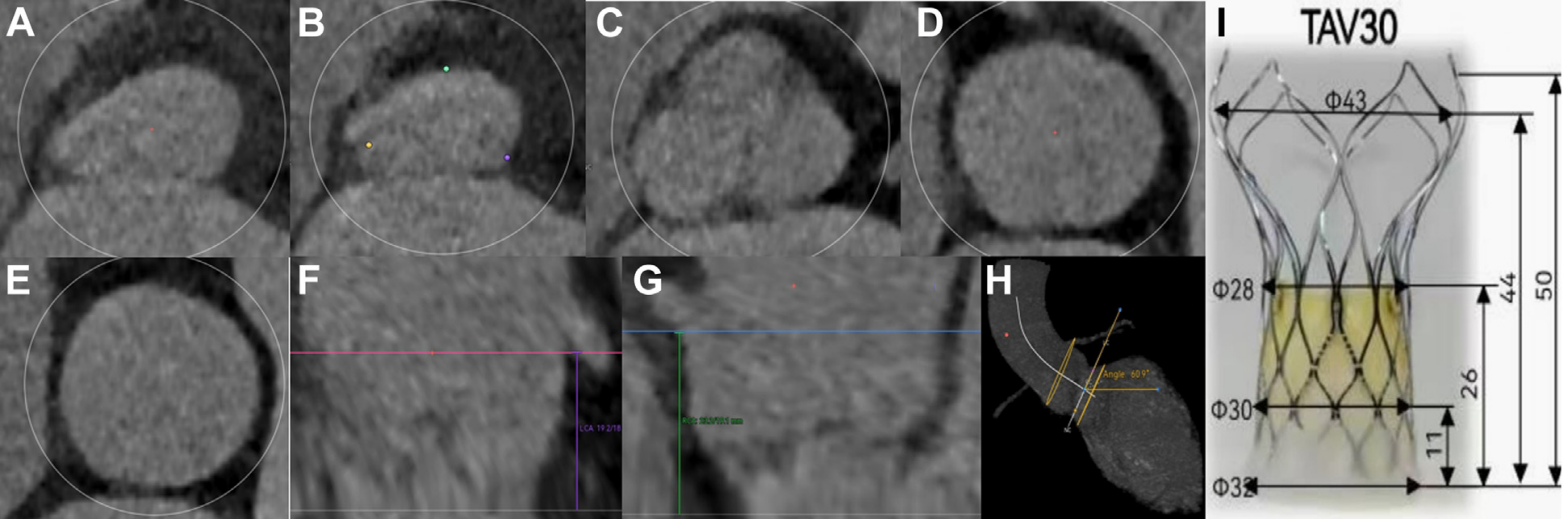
Evaluation of the Aortic Root and the Degree of Oversizing Rate by Computed Tomography Angiography (A) The left ventricular outflow tract diameter (area) was 26.7 mm; compression ratio: (323−26.7) / 32 = 16.6%. (B) The annular diameter (area) was 26.2 mm; compression ratio: (30−26.2) / 30 = 12.7%. (C) The sinus of Valsalva diameter (area) was 33.9 mm. (D) The sinotubular junction diameter (area) was 33.3 mm. (E) The ascending aorta diameter (area) was 37 mm; compression ratio: (43−37) / 43 = 14%. (F) The left coronary artery height was 19.2 mm. (G) The right coronary artery height was 19.1 mm. (H) The maximal intensity projection showed any calcification in the aortic root. (I) Radial line of the VitaFlow. TAV = transcatheter aortic valve.
